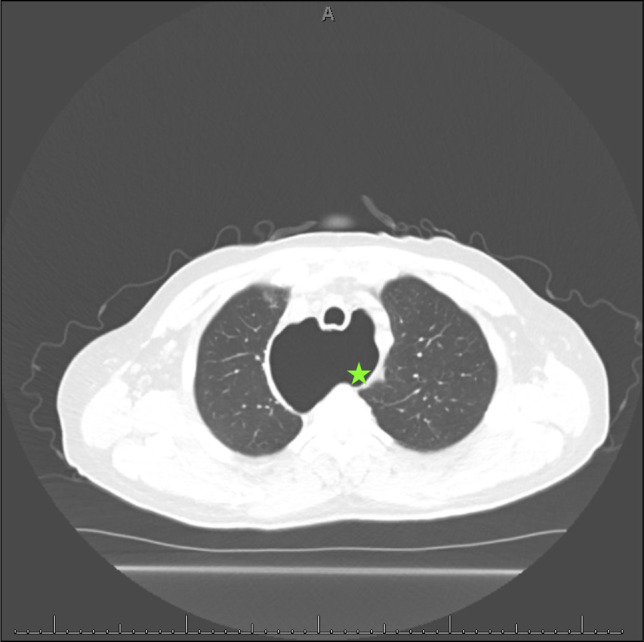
Axial computed tomography esophagram showing marked thoracic esophageal dilation (green star).
